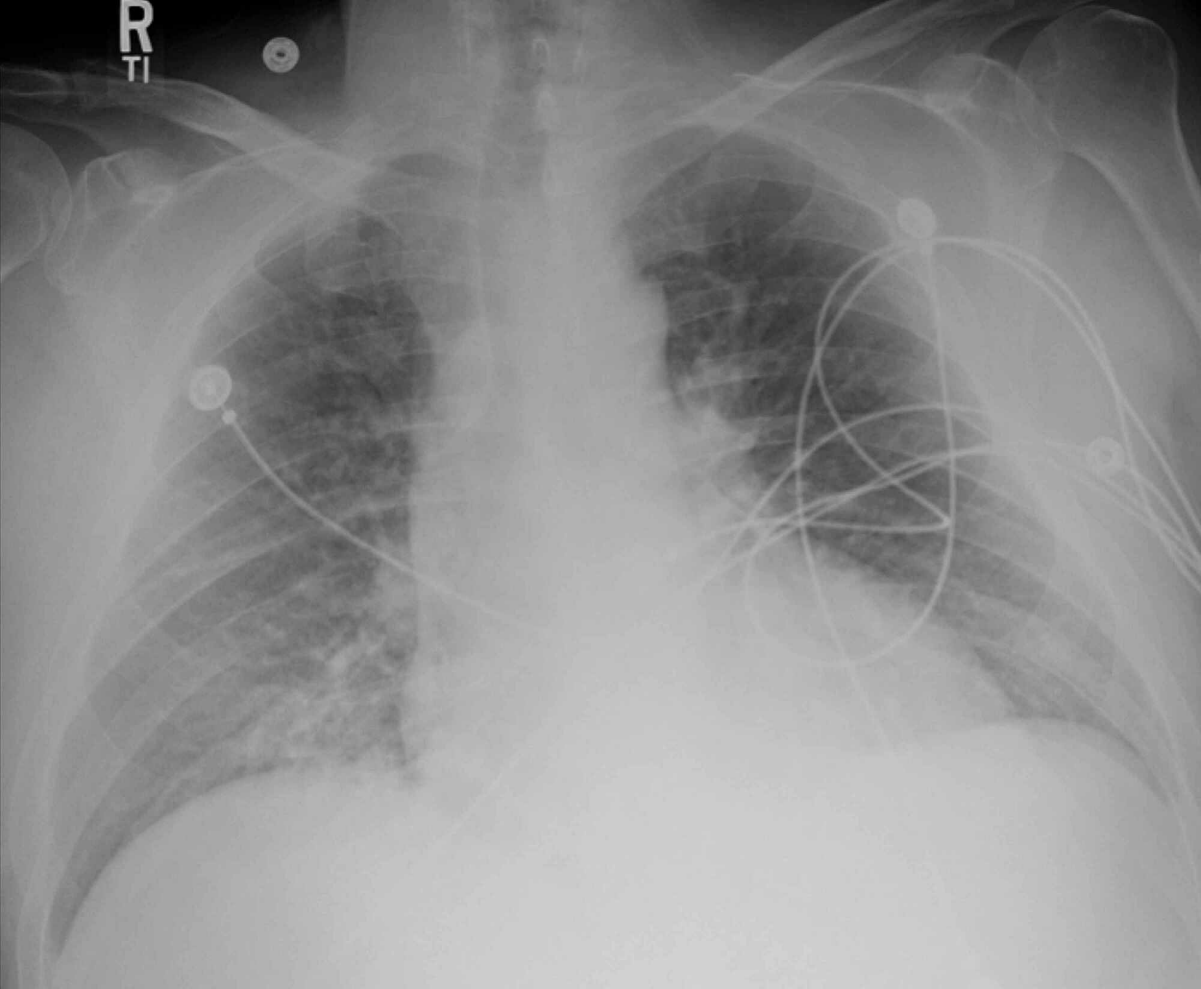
Chest X-ray showing bilateral coarsened interstitial markings and mild lower lung infiltrates
